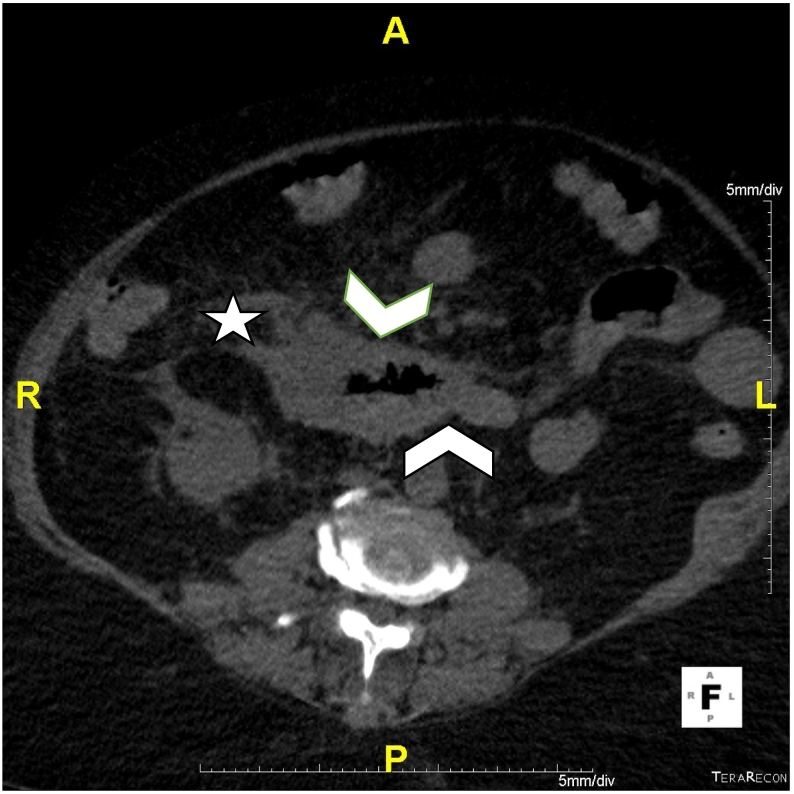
CT axial reconstruction without contrast injection showing a regular circumferential parietal thickening spontaneously hyperdense of the D2, D3 portions of the duodenum (arrowheads) with densification of the surrounding mesenteric fat (star).
